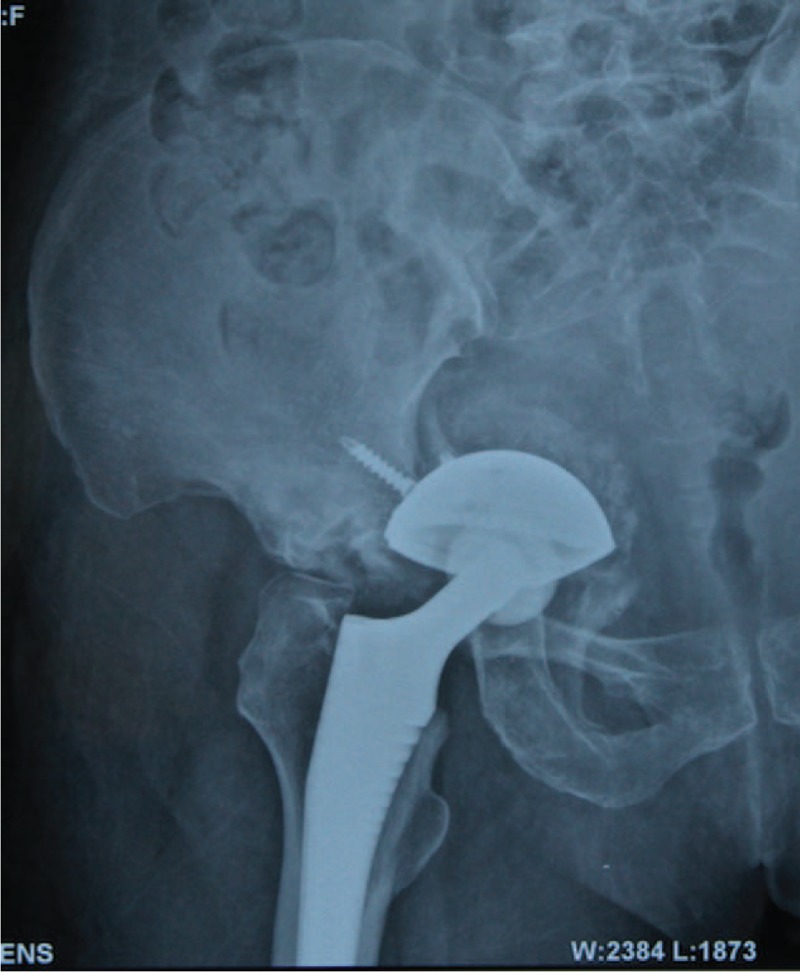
Preoperative anteroposterior radiographs of a 69-year-old female revealing a fixed but malpositioned acetabular component (uncemented).
